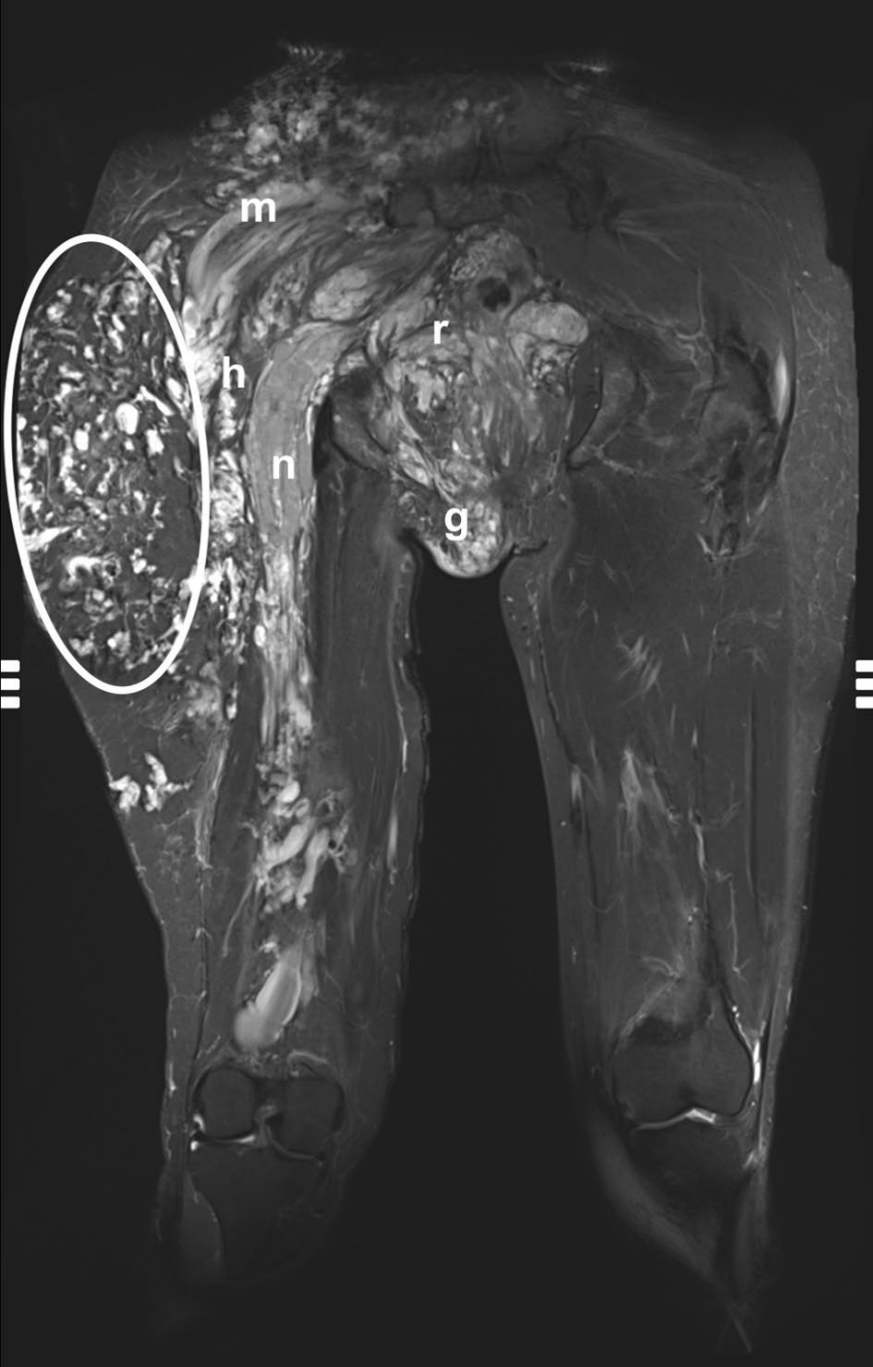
Extensive venous malformation in a 39-year old female patient. The composed T2-weighted STIR image in the coronal plane shows a subparaneurial involvement of the sciatic nerve (n), the gluteal (m) and hamstring (h) muscles, the rectum (r), the genital area (g) and the subcutis of the right thigh (ellipse).
